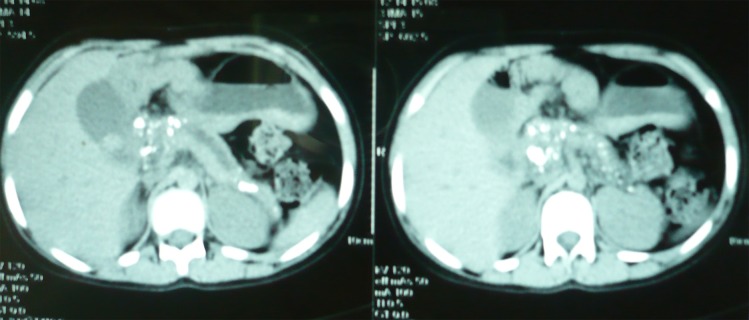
Figure 2: CT scan of abdomen revealing a dilated pancreatic duct, pancreatic calcification and gallstones
